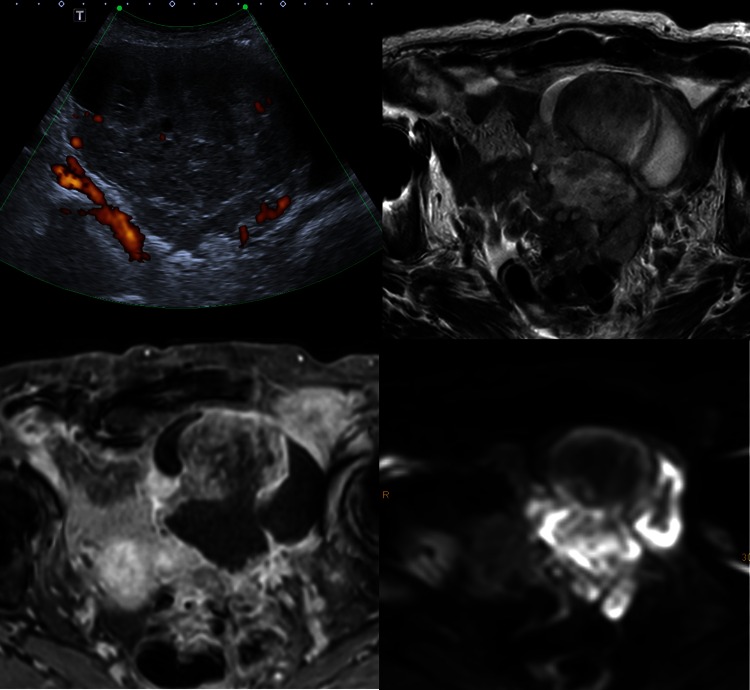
Ovarian cancer in an 87-year-old patient. Large inhomogeneous solid mass with cystic and necrotic areas and ascites in ultrasound (a) and T2W MRI (b, *arrows*). Typical findings supporting malignancy include highly vascularized solid areas (c) with restricted diffusion (d)
